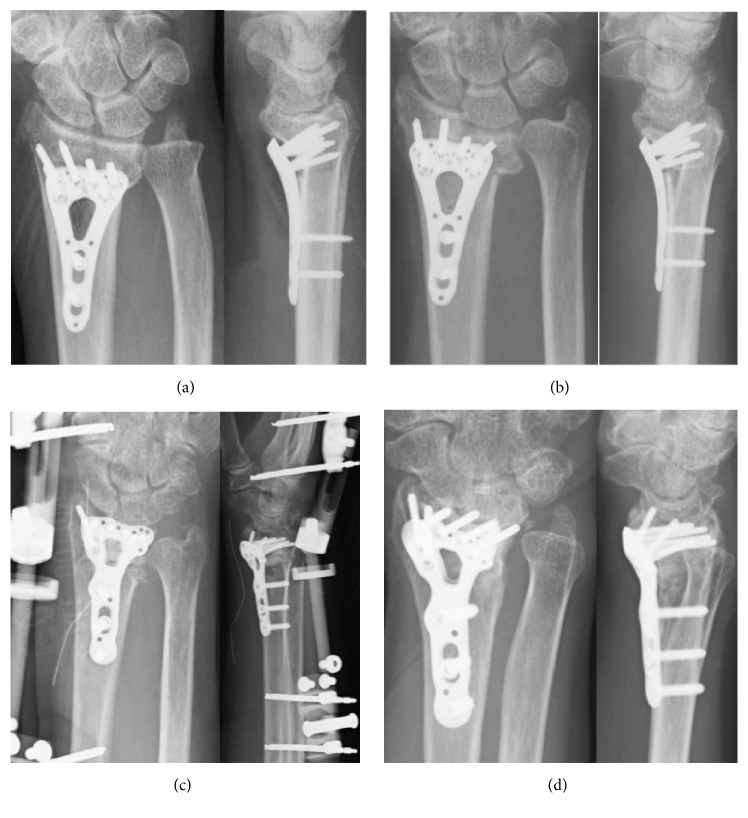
Plain radiographs of Patient 2. (a) Immediately after the first surgery. (b) Six weeks after the first surgery; redisplacement is seen. (c) Immediately after reoperation. (d) Nine months after reoperation; bone union was achieved without redisplacement.
